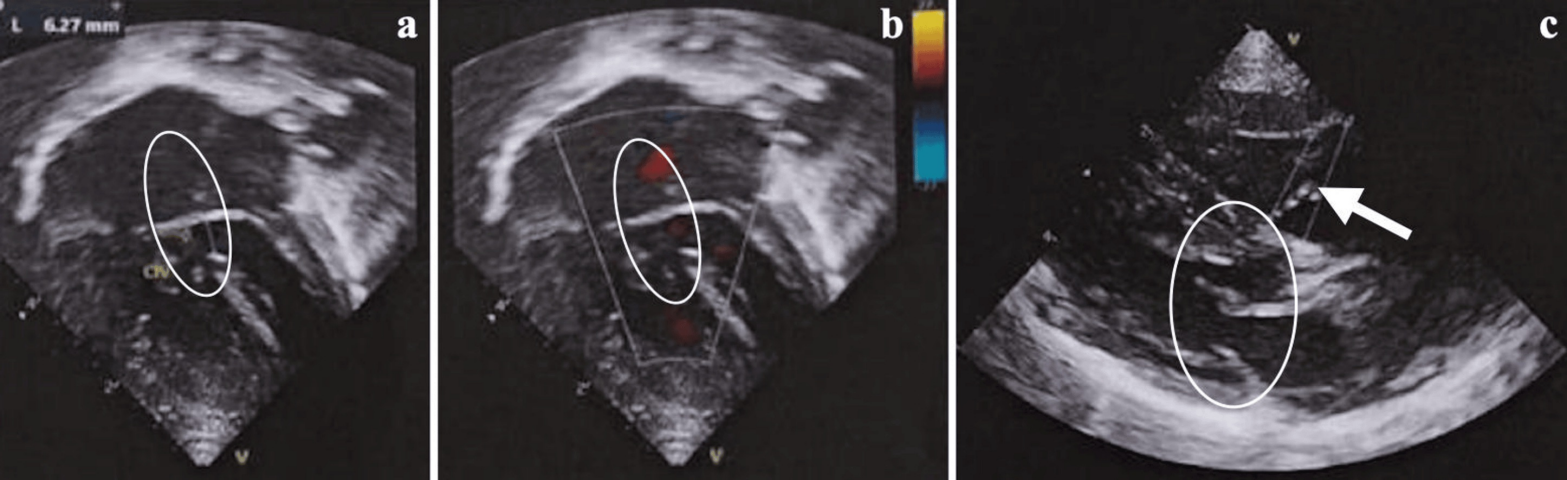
Two-dimensional echocardiography (a, b) Atrial and ventricular septal defect (circle). (c) Double-outlet right ventricle with transposition of the great vessels (white arrow). Dysplastic pulmonary valve, significant pulmonary stenosis, and patent ductus arteriosus (circle).
